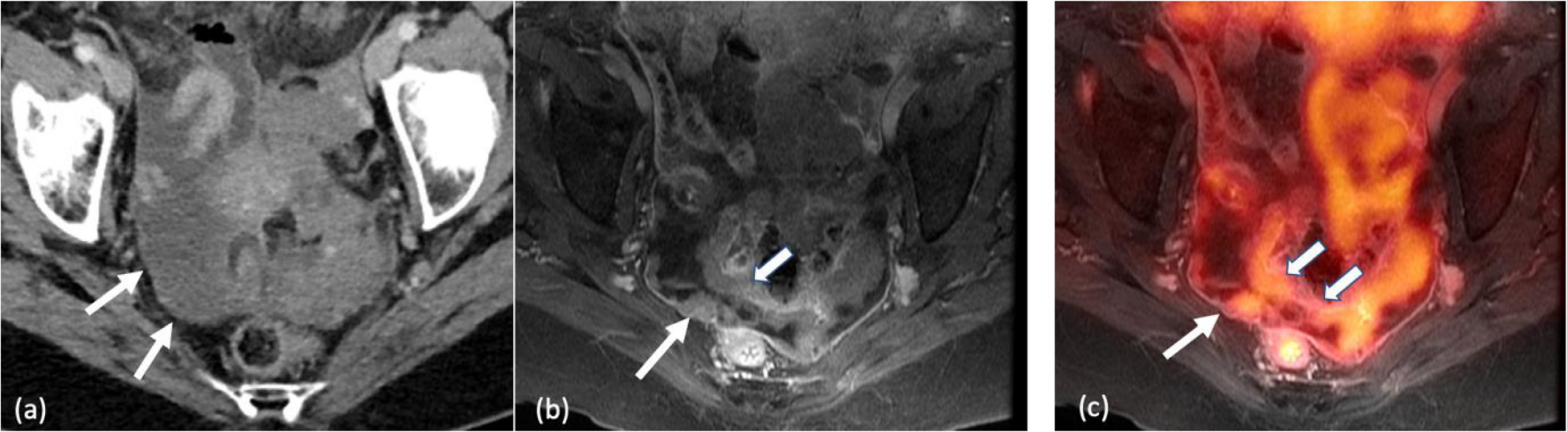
A 65-y/o female with ovarian cancer. (a) Axial contrast-enhanced CT shows ascites and peritoneal enhancement (arrows) of concern for peritoneal disease. (b) Post-contrast T1-weighted MRI shows enhancement and nodularity in the pelvis along the peritoneal lining (arrow) and thickening of the sigmoid colonic wall (fat arrow) of concern for peritoneal disease. Serosal involvement of the sigmoid colon may require colonic resection. (c) Axial fused PET/MR shows [18F]FDG-avid lesions along the pelvic peritoneal lining (arrow) and along the sigmoid colonic wall (fat arrows) representing peritoneal disease and serosal involvement of the colonic wall, which may require resection of the sigmoid colon. Images are courtesy of Priya Bhosale, MD
